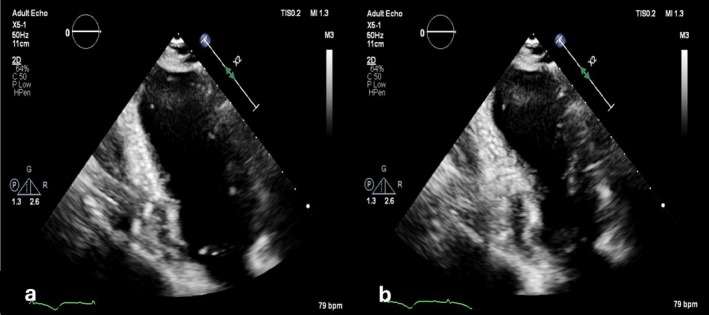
Apical two‐chamber view on 2D echocardiography showing apical akinesis (a: End diastole; b: End systole).
